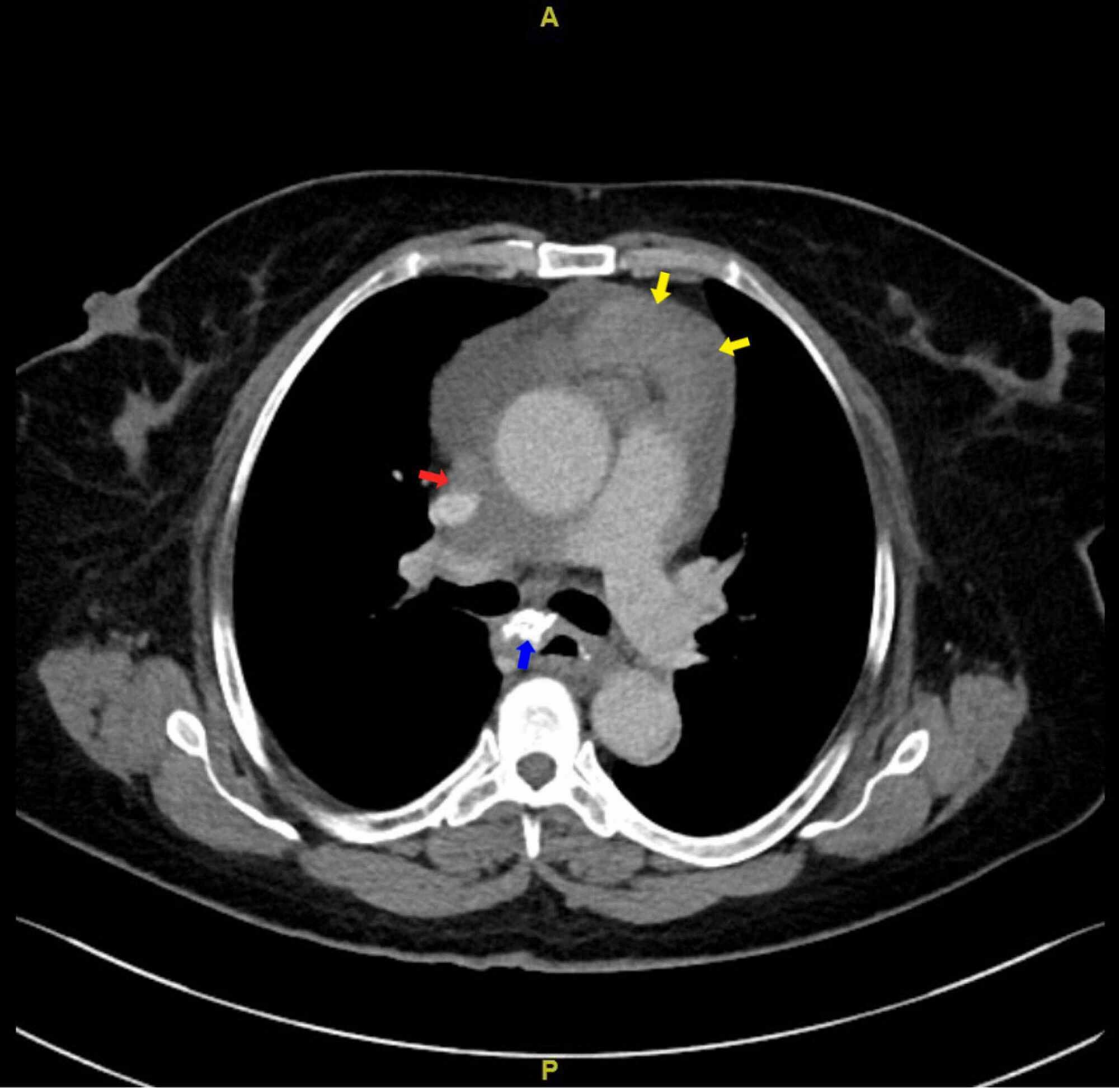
CT chest axial view: red arrow showing soft tissue density encasing SVC causing narrowing; yellow arrows showing node like mass seen in the interior mediastinum; blue arrow pointing at large calcified lymph node. Also, pericardial effusion is noted. CT: computed tomography; SVC: superior vena cava.
